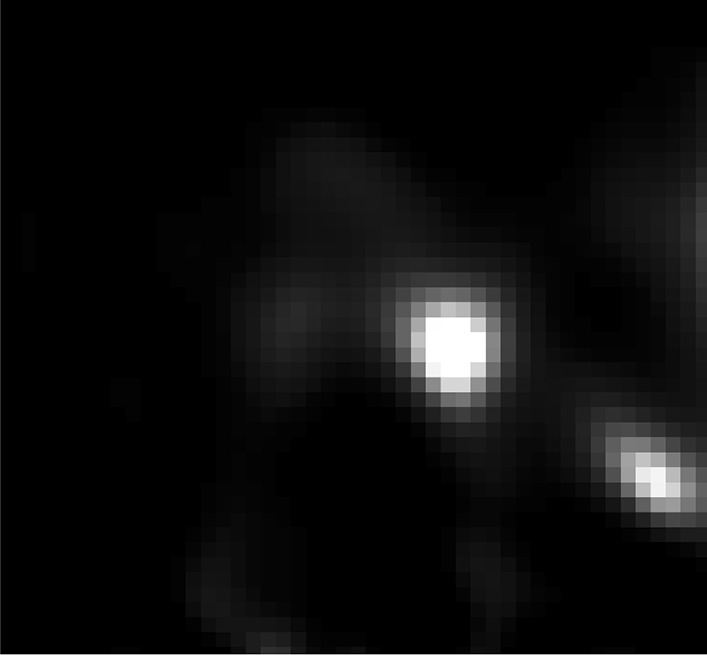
Camera fhSPECT slice, Case 7, corresponding to the red-circled portion of Fig. 9b
